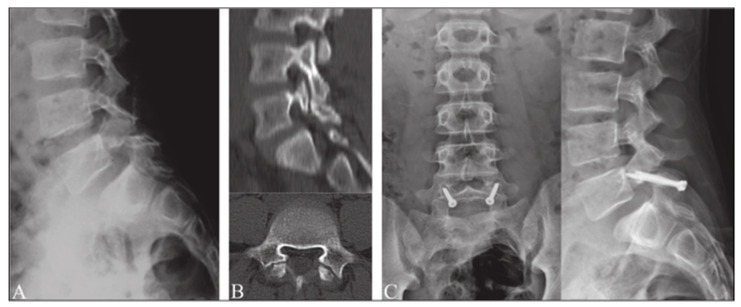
Buck’s repair technique. (A) Preoperative radiograph in lateral projection. (B) CT scan of pars defect. (C) Postoperative radiographs after surgery using Buck’s repair technique. “Reproduced with permission from Sri Vijay Anand, K.S.; Eamani, N.; Shetty, A.; Rajasekaran, S. Spondylolysis and pars repair technique: A comprehensive literature review of the current concepts. *Indian Spine J.*
